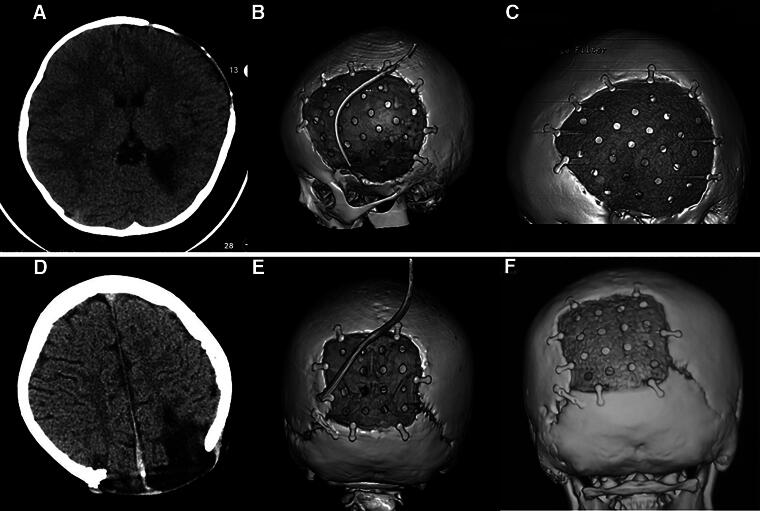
CT images of two typical patients with no bone gap expansion at the interface between cranial bone and PEEK. (A, D) Preoperative CT image. (B, E) Postoperative 3D CT image. (C, F) Follow-up 3D CT image. Patient 1: A, B, C; patient 2: D, E, F. CT, computed tomography; PEEK, polyether ether ketone.
